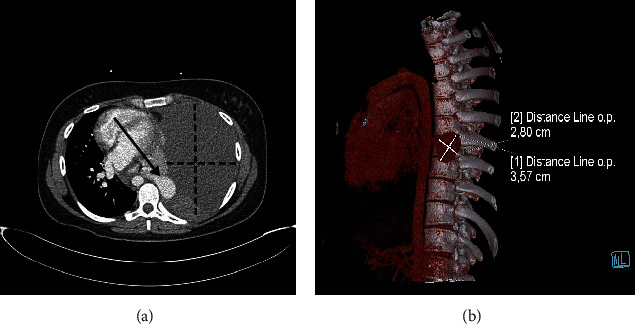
Computed tomography pulmonary angiography at 25^+4^ weeks of pregnancy of the patient presented with dyspnea on multiple occasions from 20 weeks of pregnancy. (a) Transversal plane, arrowhead pointing at arteriovenous malformation, and dotted lines indicating diameters of left haemathothorx; (b) isolated mass—arteriovenous malformation.
